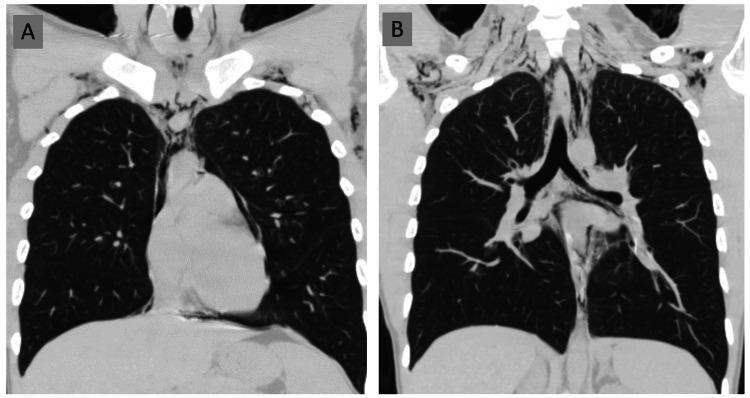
CT chest section A coronal lung window demonstrates a pneumomediastinum that extends into the superior mediastinum [A] and subcutaneous emphysema tracks along the superior aspect of the thoracic wall and neck soft tissues [B]. No pneumothorax is visible.
